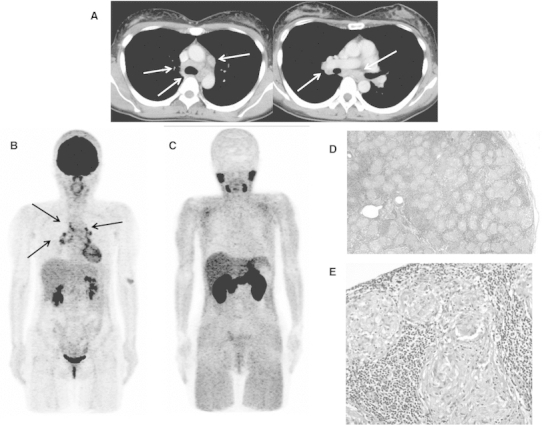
A case of sarcoidosis. Forty-four-year-old asymptomatic female patient. She had been followed as an outpatient after complete remission (more than 1 year previously) of malignant abdominal lymphoma (post-chemotherapy). She had no history of sarcoidosis or uveitis. The patient underwent chest CT scan (a), which revealed multiple nodules (*arrows*) in the middle mediastinum. ^18^F-FDG PET (b: MIP image) showed high accumulation in these lesions (SUVmax = 6.0 for the highest uptake in right hilar lesion), but there was no other lesions in the body. ^11^C-MeAIB PET (c: MIP image) showed no accumulation in these lesions (SUVmax = 1.8, with the highest uptake in the mediastinum). Thoracoscopic biopsy revealed sarcoidosis (d: low magnification, e: high magnification)
